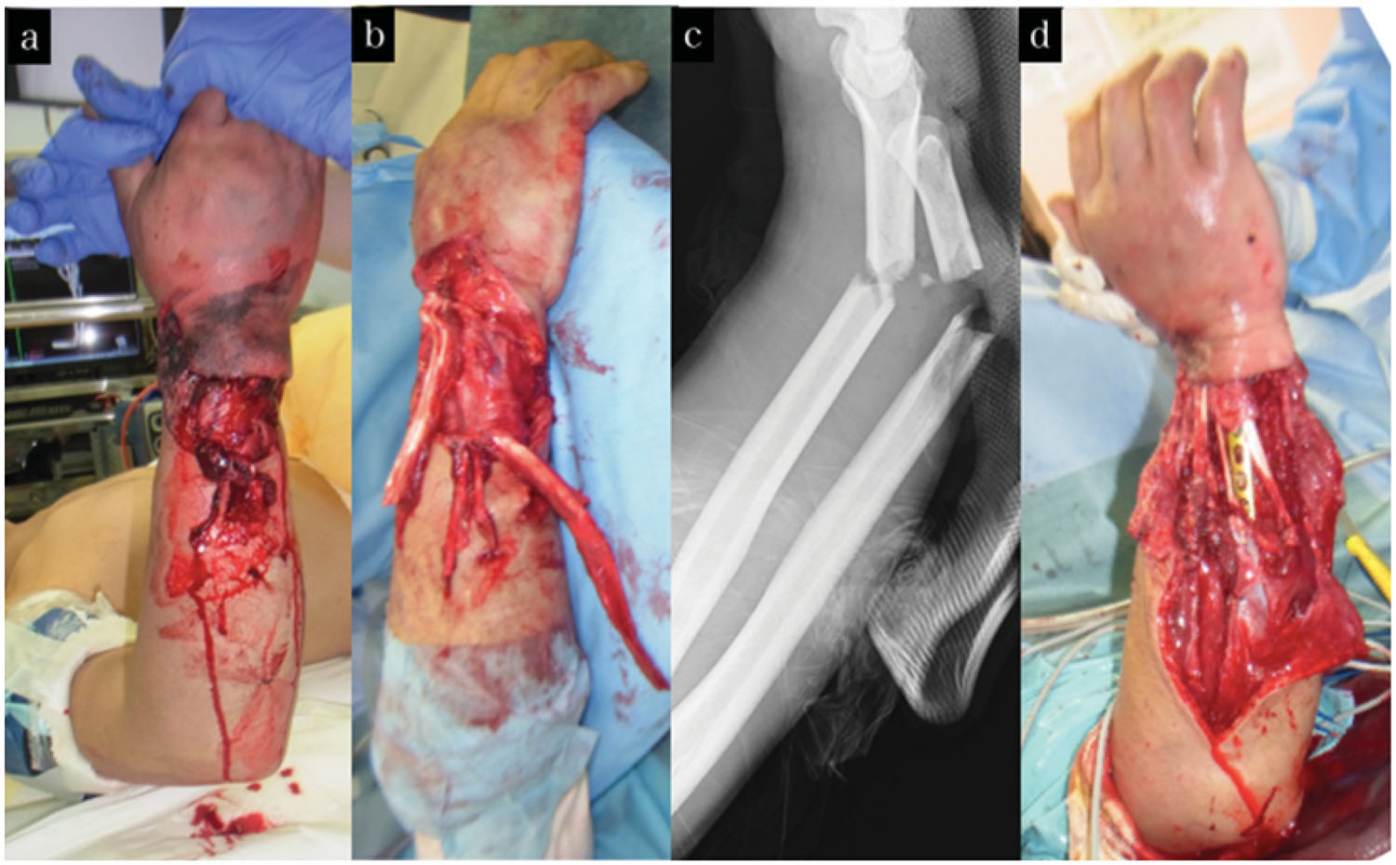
Treatment course of case 2. (a,b) Appearance on arrival at our hospital. (c) X-ray on arrival at our hospital. (d) Appearance after tendon reconstruction using pull in suture and osteosynthesis.
